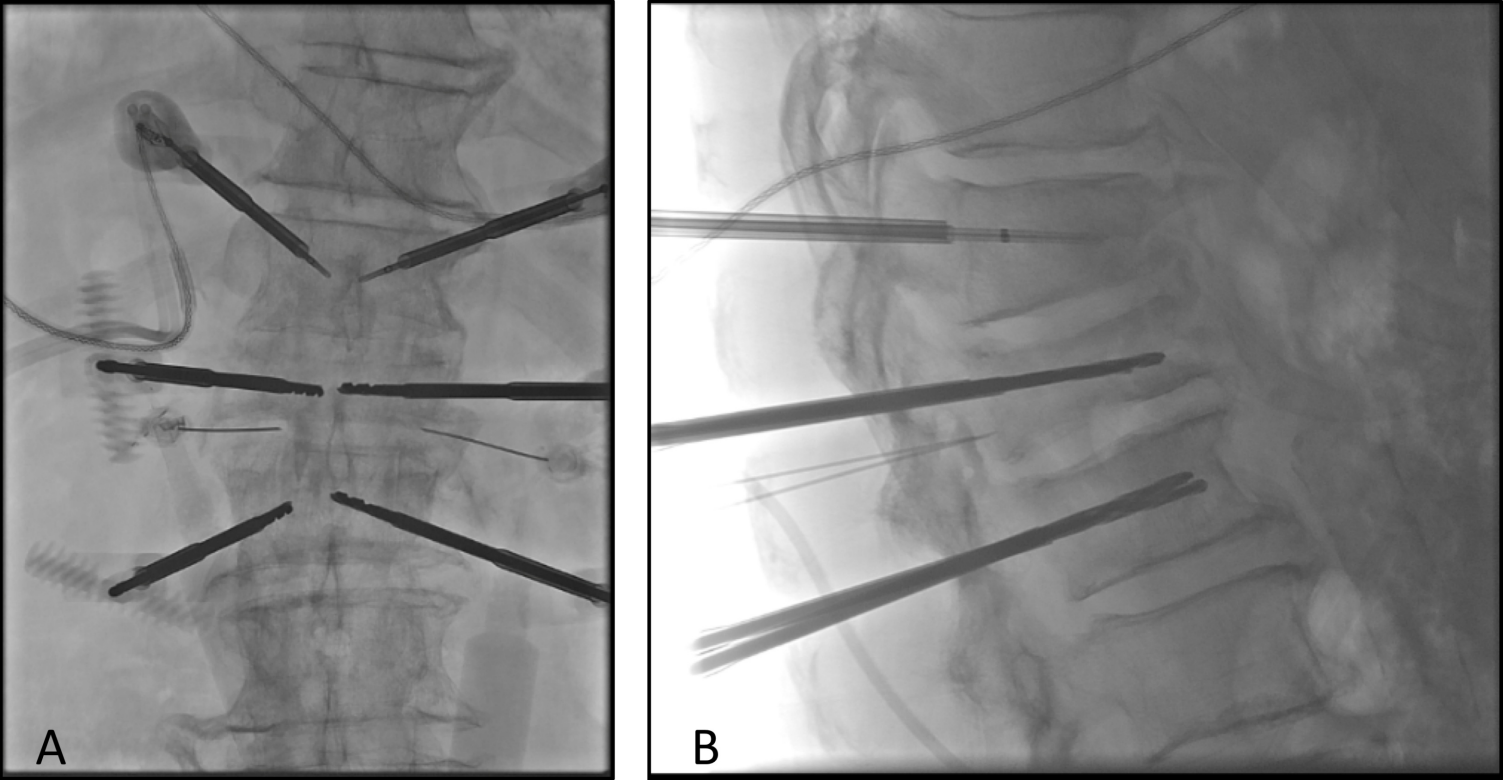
Intraoperative AP (A) and lateral (B) radiographic views
